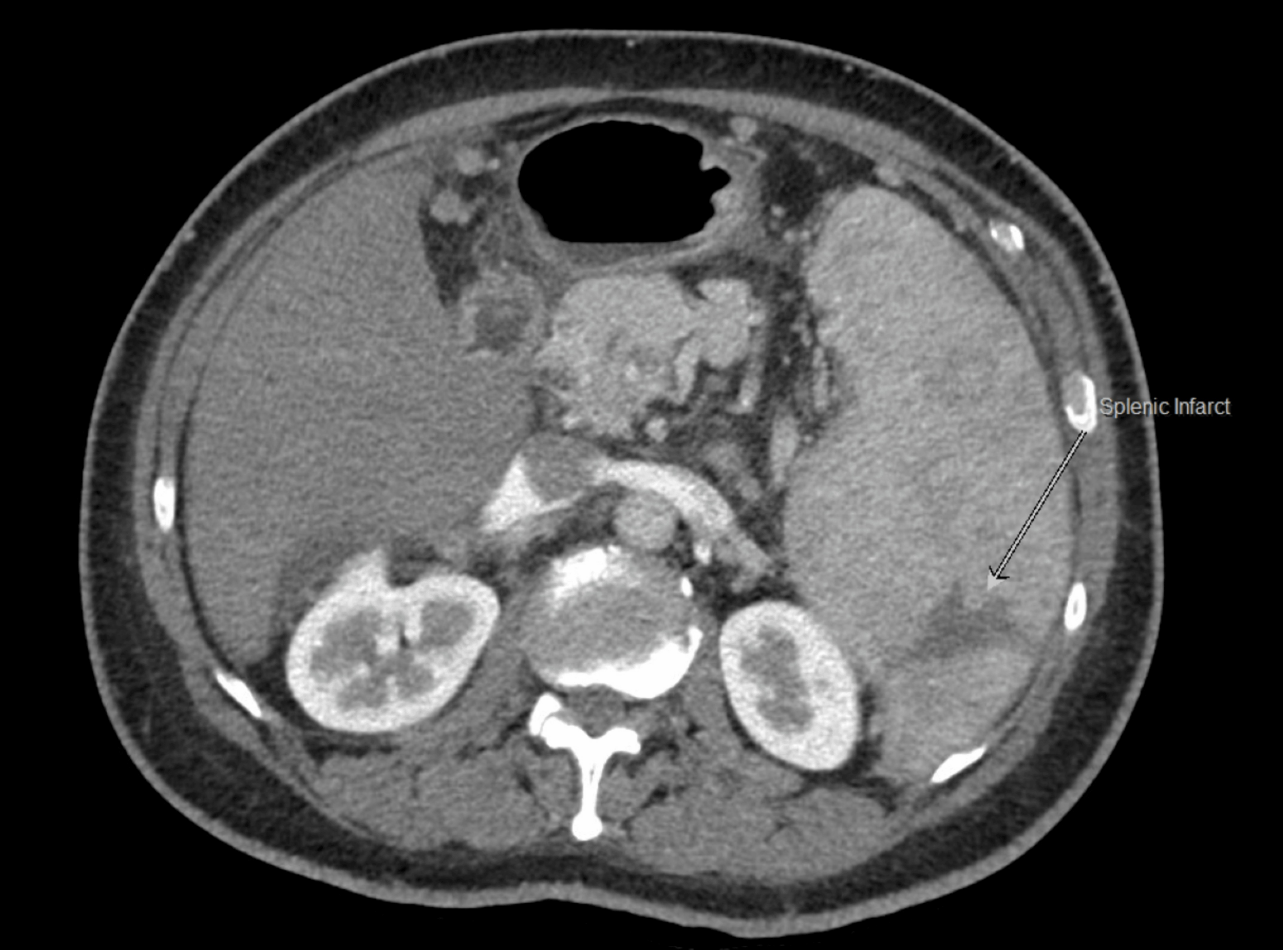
Abdominal CT image revealed a wedge-shaped splenic infarct (as indicated by the grey arrow)
